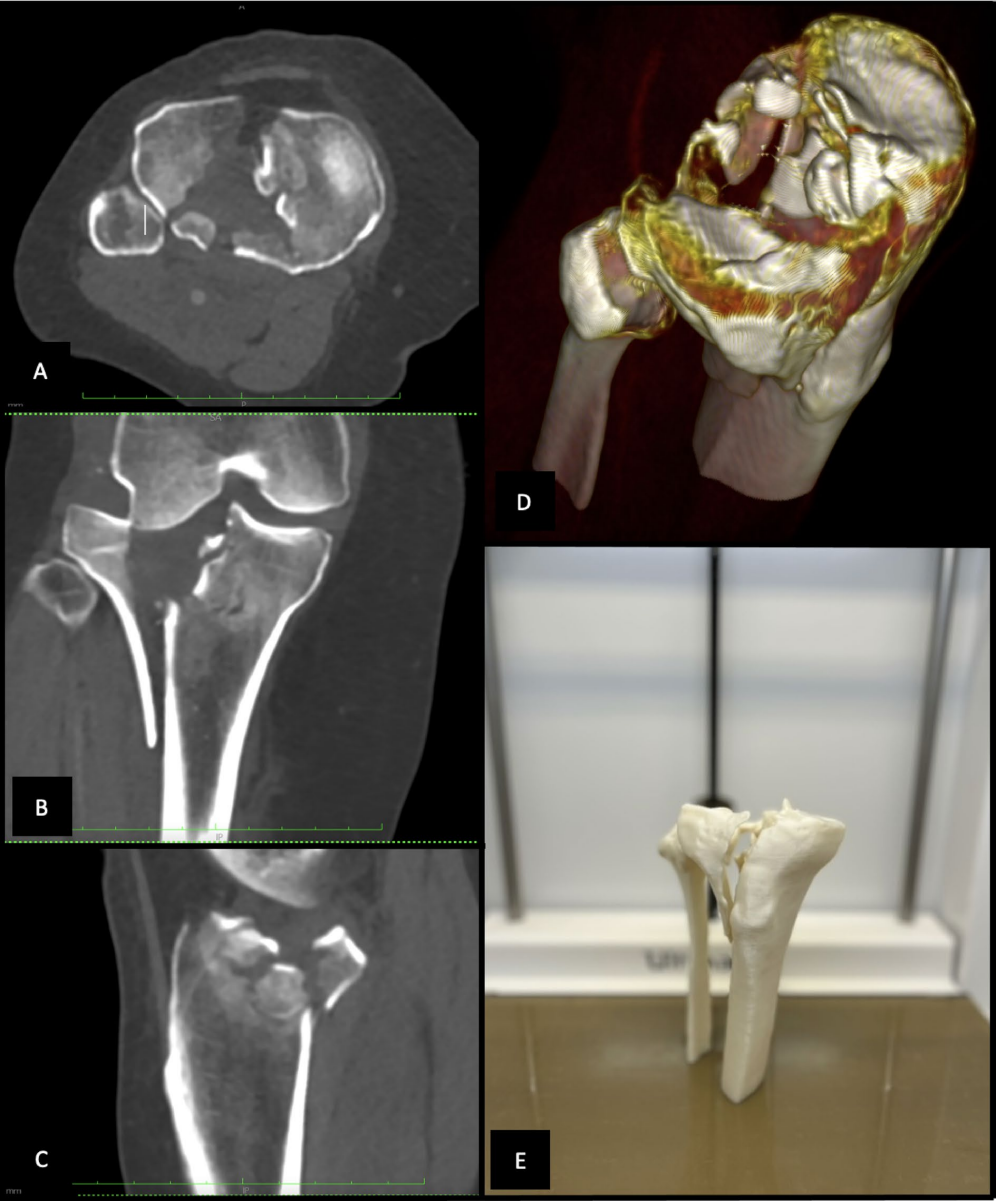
Multifragmentary lateral tibial plateau fracture shown as a CT-scan (A–C), a 3D volumetric reconstruction (D) and as a 3D-printed model (E).
